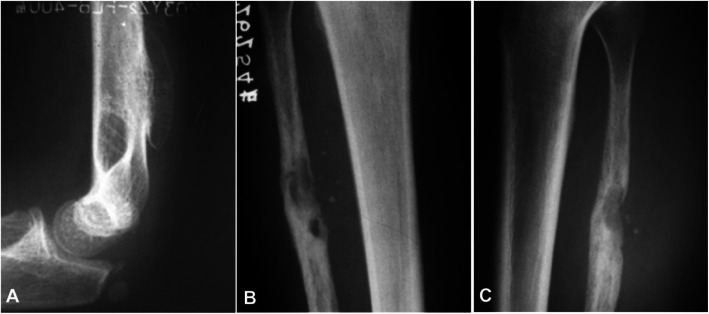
Periosteal hemangioma. a. Plain radiograph of the lateral view of distal humerus showed an expansile lesion at the lateral humerus with radial bone crest perpendicular to the bone cortex inside the lesion. Coarse ridges were also seen inside the lesion. b & c. Pain radiograph of the anteroposterior view of the middle and upper fibula revealed that the middle and upper segments of the right fibula were enlarged with irregular osteosclerosis and low-density lesions inside. Local bone cortex was depressed, forming a pressure trace, and punctate calcification was demonstrated inside the adjacent soft tissue
